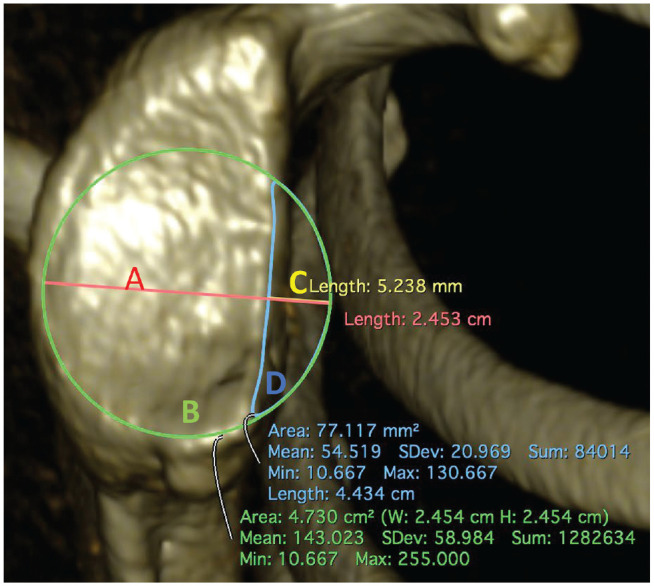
En face view of the glenoid on a 3-dimensional CT. The diameter (*A*; red) and the area (*B*; green) of the BFC positioned on the inferior aspect of the glenoid were determined. The extent of the defect was determined 1-dimensionally by assessing the defect diameter (*C*; yellow) in relation to the BFC diameter (*A*) and 2-dimensionally by assessing the defect area (*D*; blue) in relation to the BFC area (*B*).
